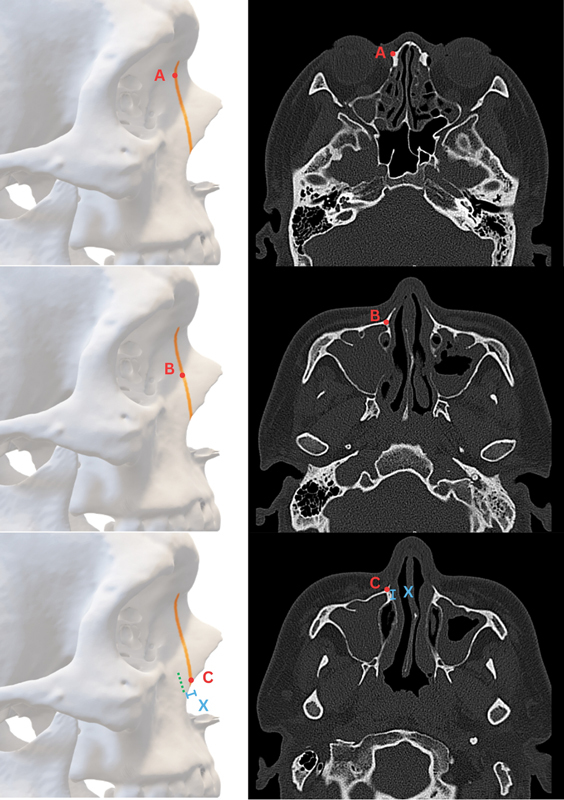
The low-to-low osteotomy path was defined using three CT points: It began at the lacrimal fossa (corresponding to the area of the medial canthal ligament) (A) and passed through the widest point of the nose (B). The osteotomy line was projected to a third point over the most caudal nasal bone (C), and the distance from this third point to the inferior turbinate was then measured (X). (A) Lacrimal fossa, (B) the widest point of the nose at the frontal process of the maxilla, (C) the projected osteotomy line over the most caudal nasal bone, and (X) distance from the projected osteotomy line to the inferior turbinate.
